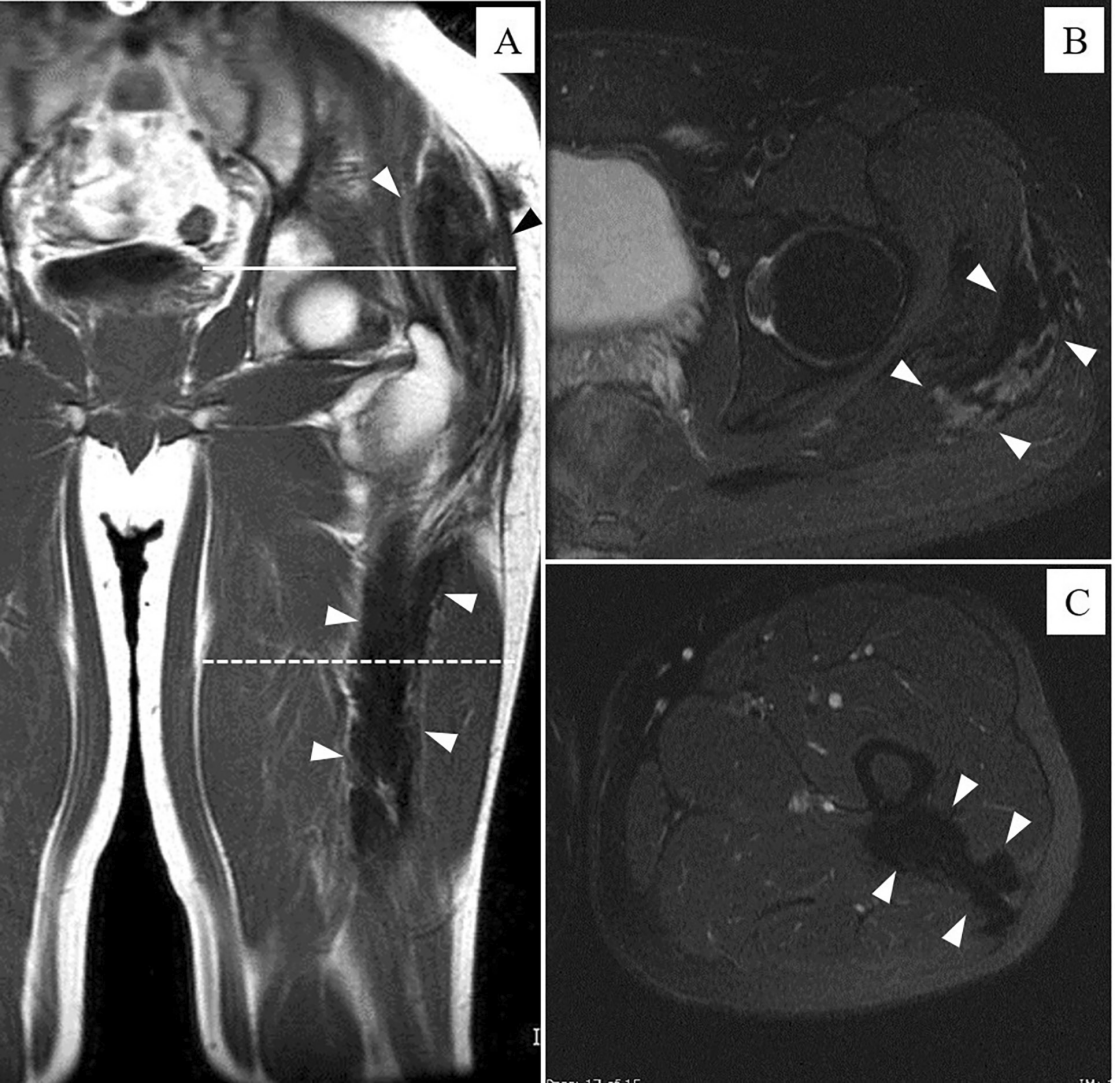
Magnetic resonance imaging analysis. Homogeneously low‐intensity lesion on the T1‐weighted and short TI inversion recovery images of the left gluteus maximus and gluteus medius muscles. The tumor shows increasing low signal area and edema of the muscle 3 years after CIRT. The tumor size decreased. The tumor is indicated by arrowheads. (A) T1WI coronal sequence (gluteus muscle slice, femoral muscle slice). (B) Short TI inversion recovery axial sequence (gluteus muscle slice). (C) Short TI inversion recovery axial sequence (femoral muscle slice).
